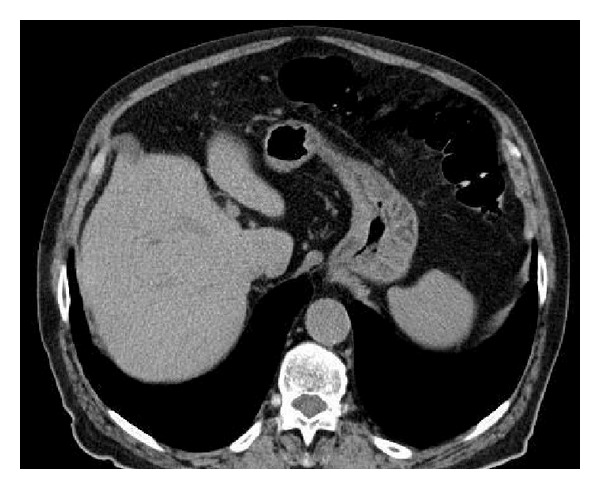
Transverse CT image showed the complete disappearance of gas in mesenteric vein and portal venous system, 24 hours after the CTC.
